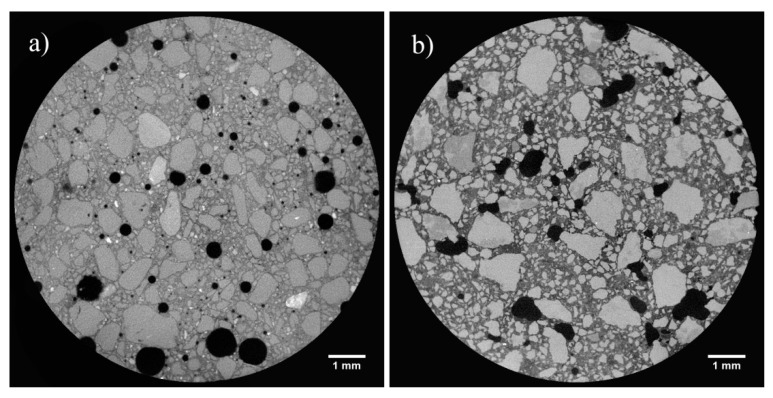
Slices of X-ray tomography of samples (a) NQ and (b) NM.
